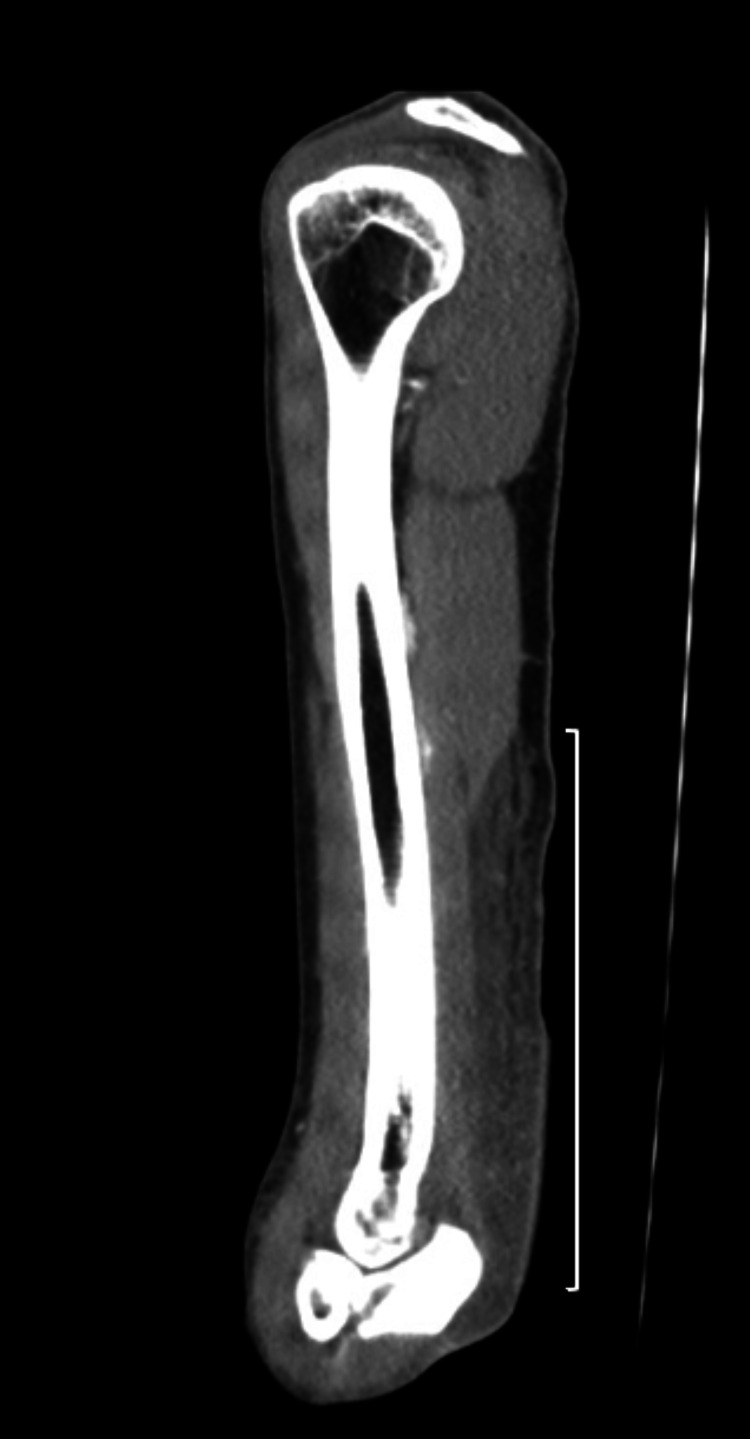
A CT scan of the left upper extremity in the sagittal plane showing subcutaneous thickening with no evidence of defined fluid collection, abscess, or subcutaneous gas extending from the level of the mid left humerus posteriorly and medially through the left elbow as outlined by the bracket on the right
